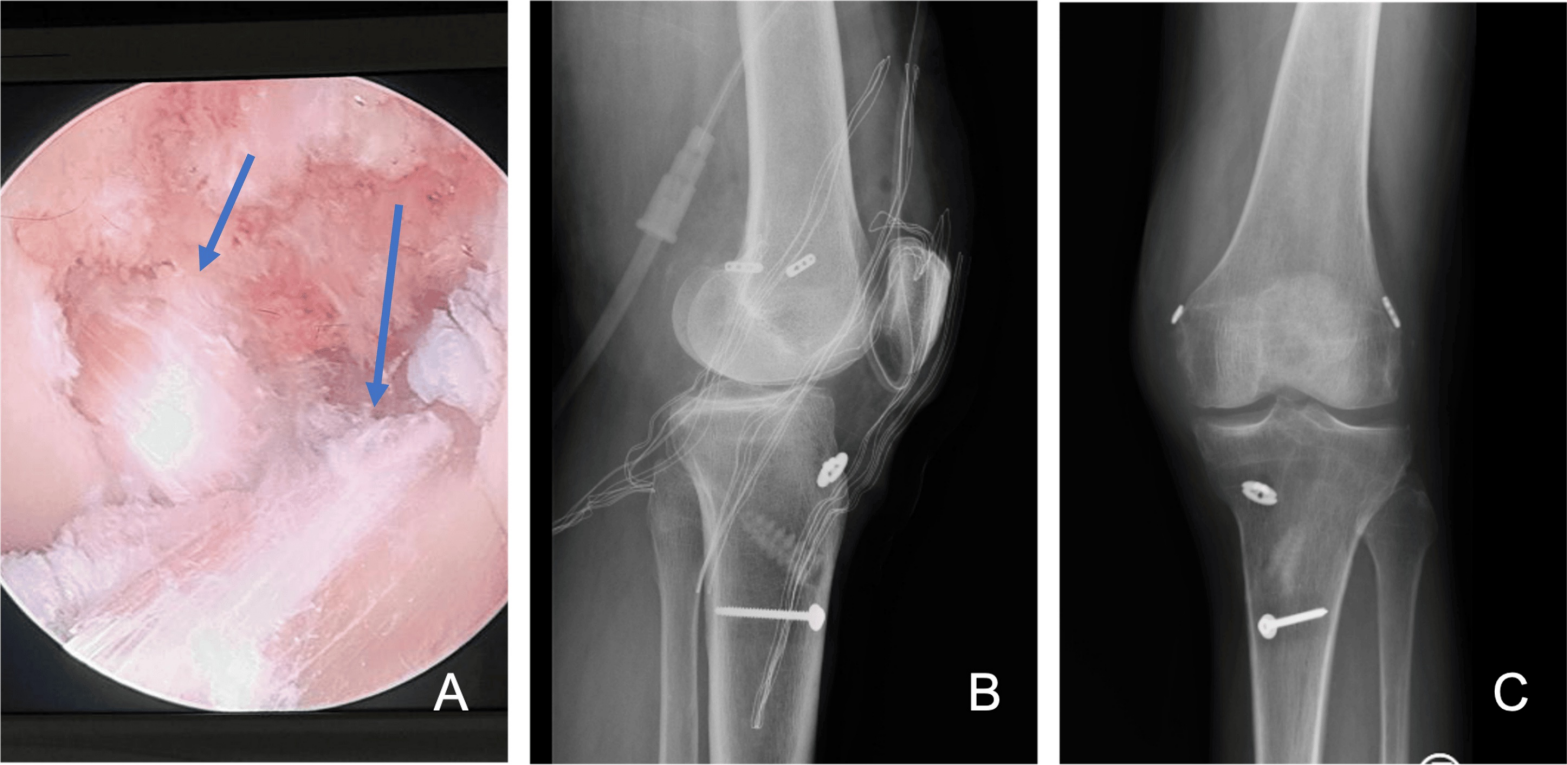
Arthroscopic and postoperative imaging following bicruciate reconstruction Intraoperative and postoperative imaging following second-stage bicruciate ligament reconstruction. A: arthroscopic view demonstrating completed anterior and posterior cruciate ligament reconstructions (marked by blue arrows); B: anteroposterior; and C: lateral radiographs of the left knee confirming appropriate tunnel positioning and graft fixation.
